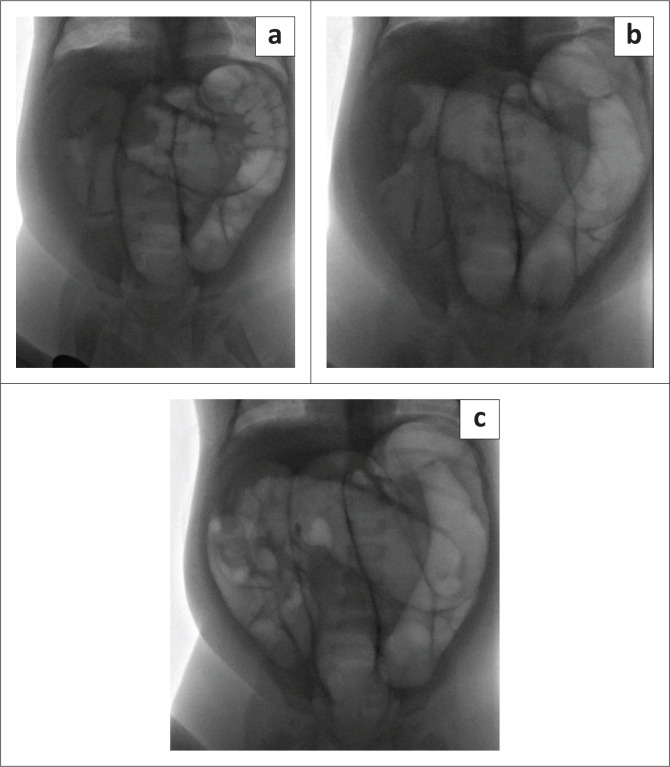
(a–c): A case of an intussusception that was successfully reduced with fluoroscopy-guided air enema reduction (FGAR) after an ultrasound was done, which confirmed an ileocolic intussusception with a ‘target sign’ in the midline upper abdomen. (a) Initially, a soft tissue mass ‘meniscus sign’ can be appreciated within the proximal transverse colon, with minimal air in the small bowel and no signs of perforation. (b) After initial low starting pressure enema at 80 mmHg, one can appreciate slight movement of the ‘meniscus sign’ and/or soft tissue mass at the hepatic flexure. (c) Absence of the ‘meniscus sign’ within the colon or small bowel, with air appreciated within the small bowel after successful reduction at 100 mmHg.
